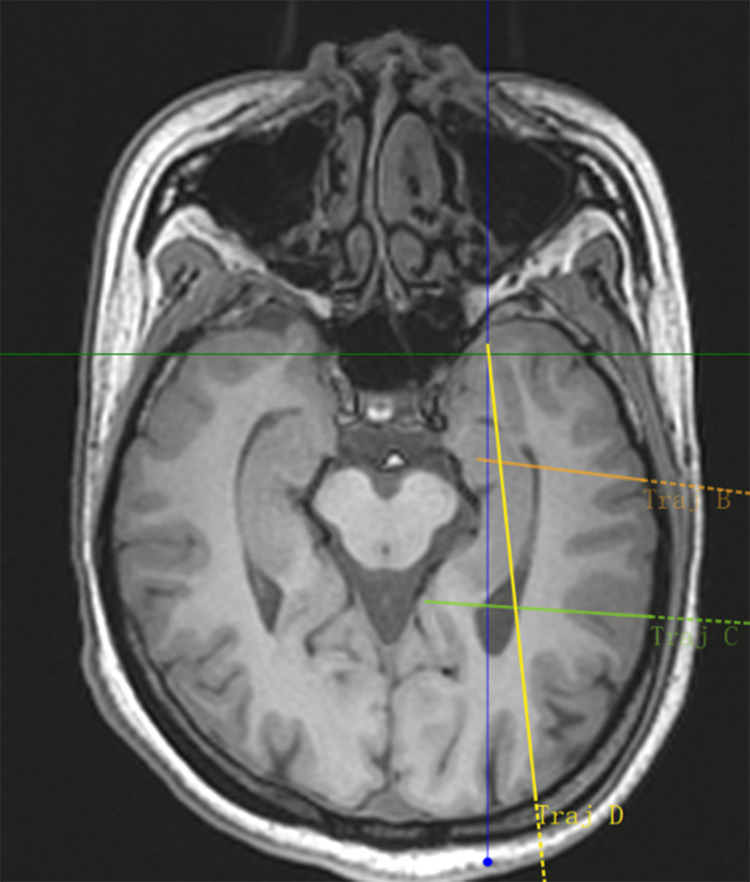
MRI T1 sequence transverse view.
